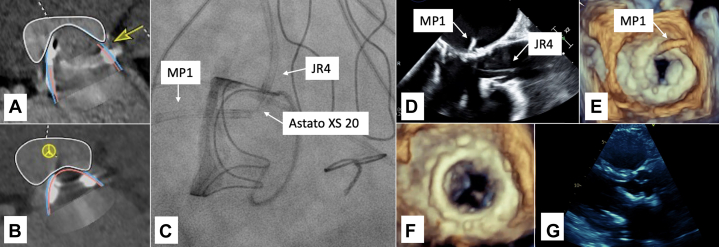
Imaging for Case 2 (A) CTA of neo-LVOT 227 mm^2^ without LAMPOON. The prior bioprosthetic mitral valve (yellow arrow). (B) CTA rendering of neo-LVOT 337 mm^2^ with LAMPOON. (C) Fluoroscopy of tip-to-base LAMPOON. (D) Two-dimensional TEE of tip-to-base LAMPOON. (E) Three-dimensional TEE of tip-to-base LAMPOON. (F) Three-dimensional TEE after tip-to-base LAMPOON. (G) TTE parasternal long-axis view showing LVOT after TMVR with 29-mm Edwards S3, with LVOT peak gradient 3 mm Hg. TMVR = transcatheter mitral valve replacement; TTE = transthoracic echocardiogram; other abbreviations as in Figure 1.
